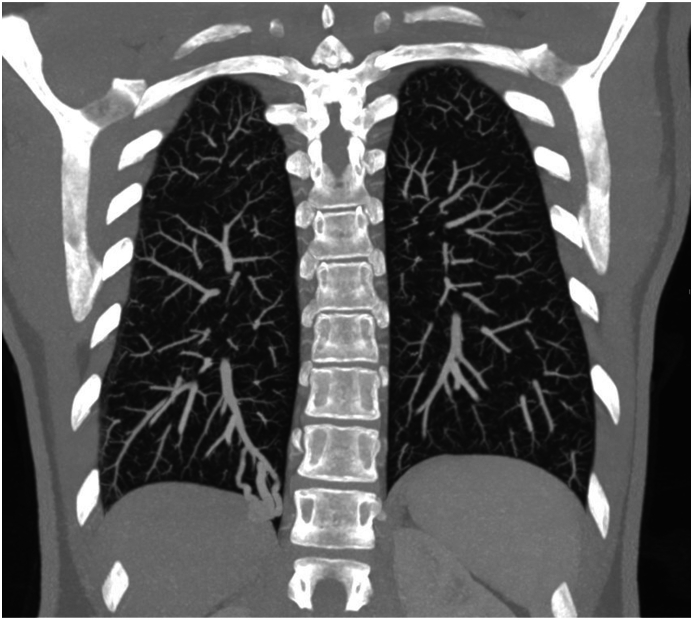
Coronal Maximal Intensity Projection (MIP) Computed Tomography image of the chest of a 39-year-old female with recurrent transient ischemic attacks, a pulmonary arteriovenous malformation is present in the right lower lobe.
